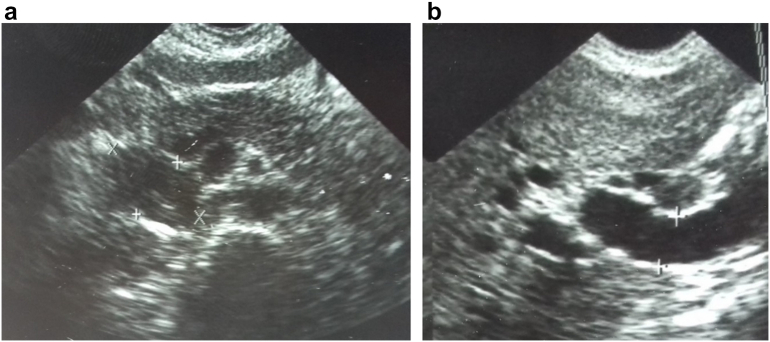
(A, B): Ultrasonography revealed a round, low-echoic mass, responsible for dilated biliary and pancreatic ducts.
